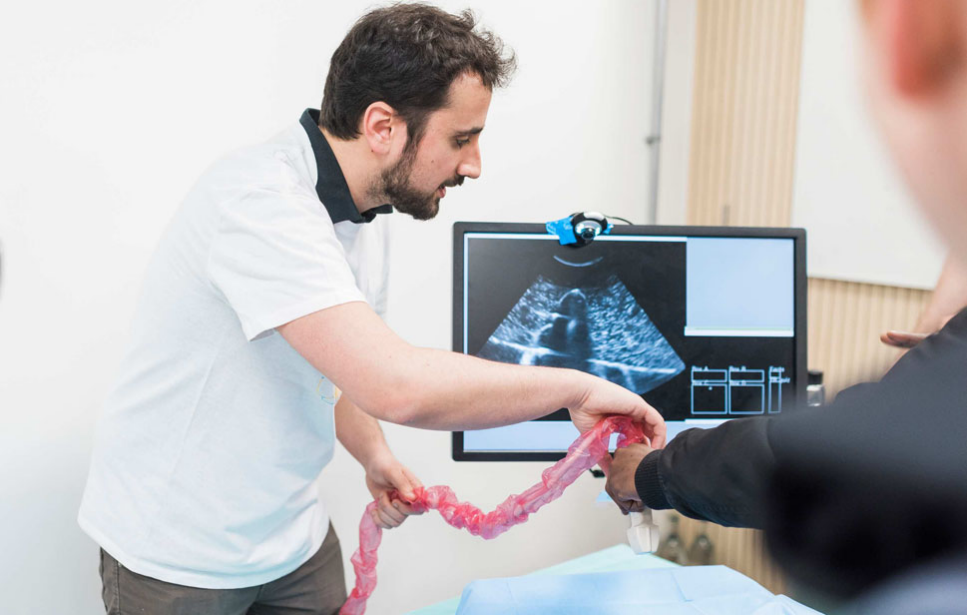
SnappySonic in use at the Science of Surgery public engagement event. An obsolete ultrasound probe is tracked using OpenCV’s ArUco marker libraries. The position of the probe is used to show an image from a pre-recorded ultrasound buffer, giving the appearance of live acquisition. Image by James Tye
